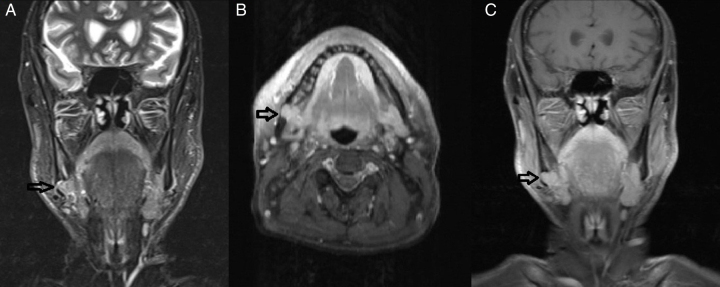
The magnetic resonance images of the lobulated mass of right submandibular region (black arrows) in T1A sections (A), and in T2 fat suppressed sections (B and C).
